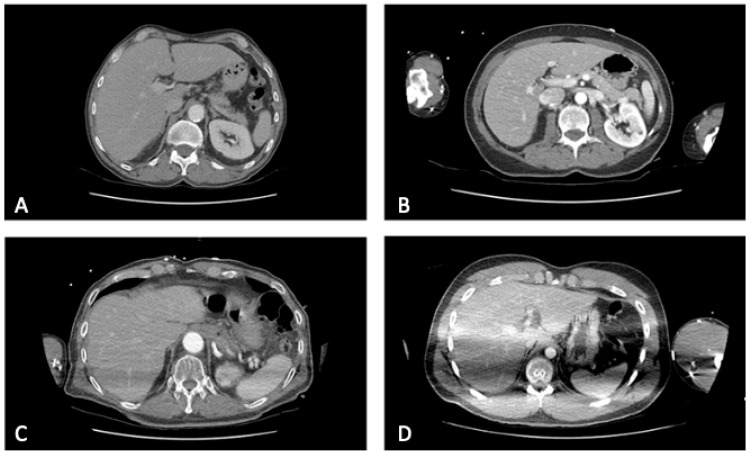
Axial contrast-enhanced CT images of the upper abdomen representing examples of the evaluation of the subjective image quality: (A) no artifacts resulting from the arms (score = 1), (B) artifacts without impairment of the image quality (score = 2), (C) artifacts with a moderate impairment (score = 3), (D) massive artifacts (score = 4).
